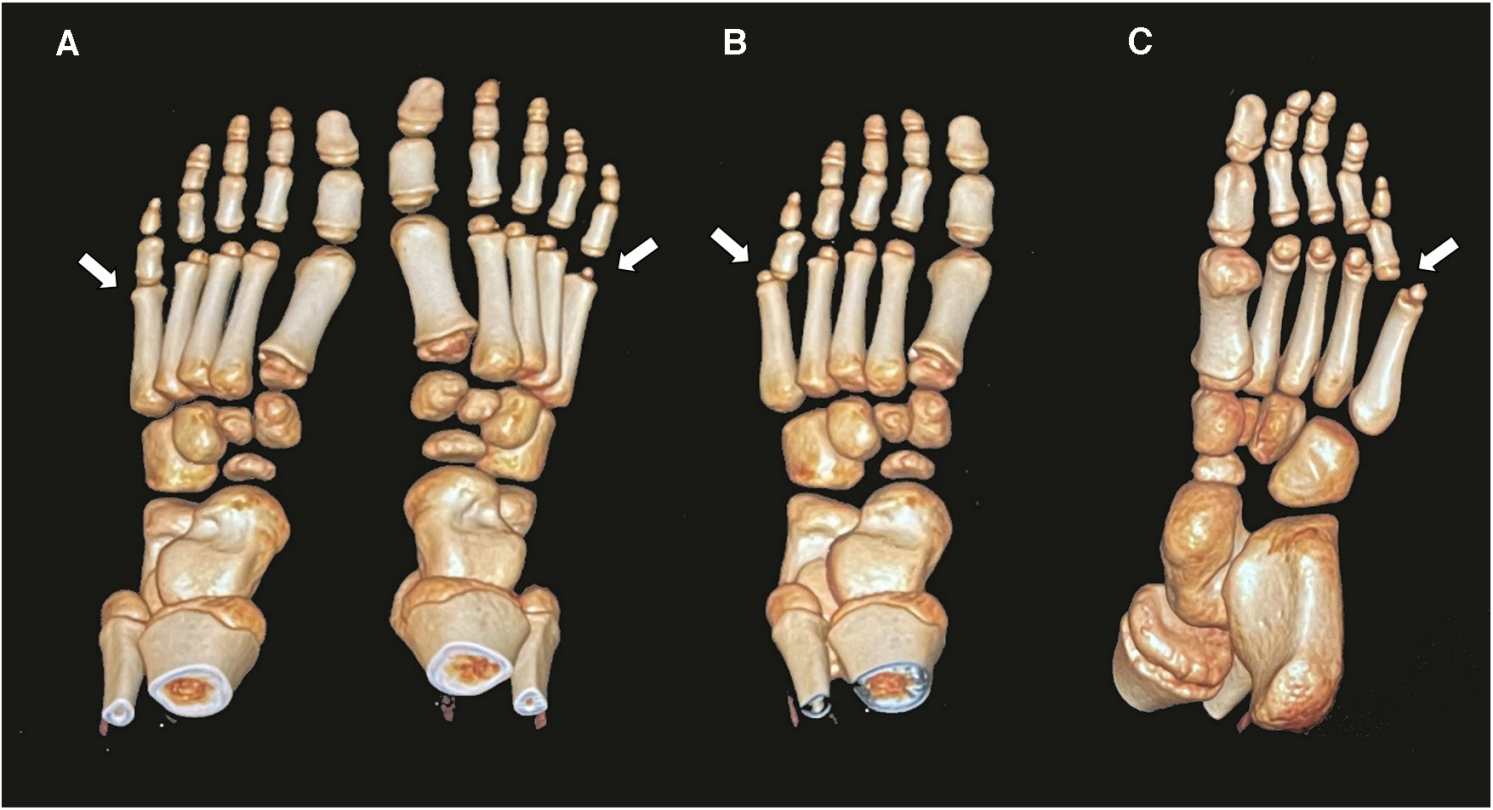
Three-dimensional computed tomography shows the dislocation of the MTPJ and normal MTPJ (A); the image in front aspect (B); the image in planta pedis (C).
